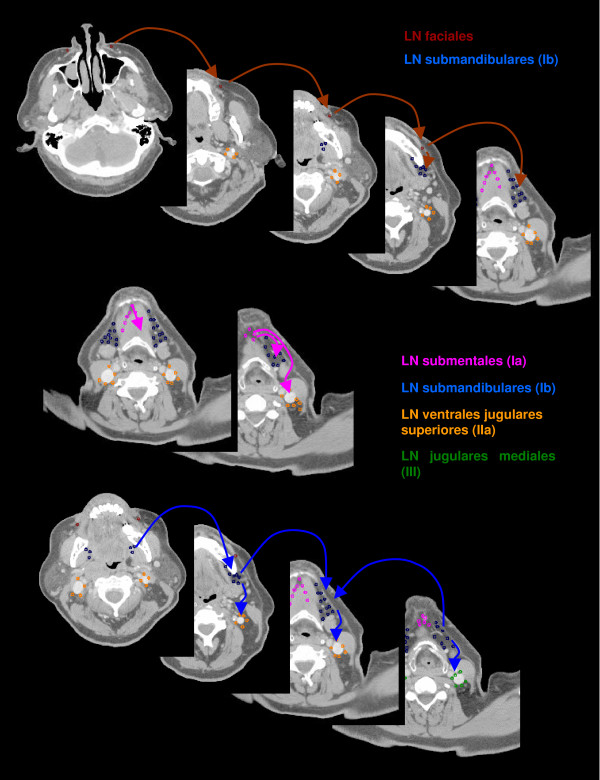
Lymph regions and drain contoured in transversal CT slices: LN buccales (brown), LN submentales (pink) and LN submandibulares (dark blue) [1.8 cm slice thickness].
